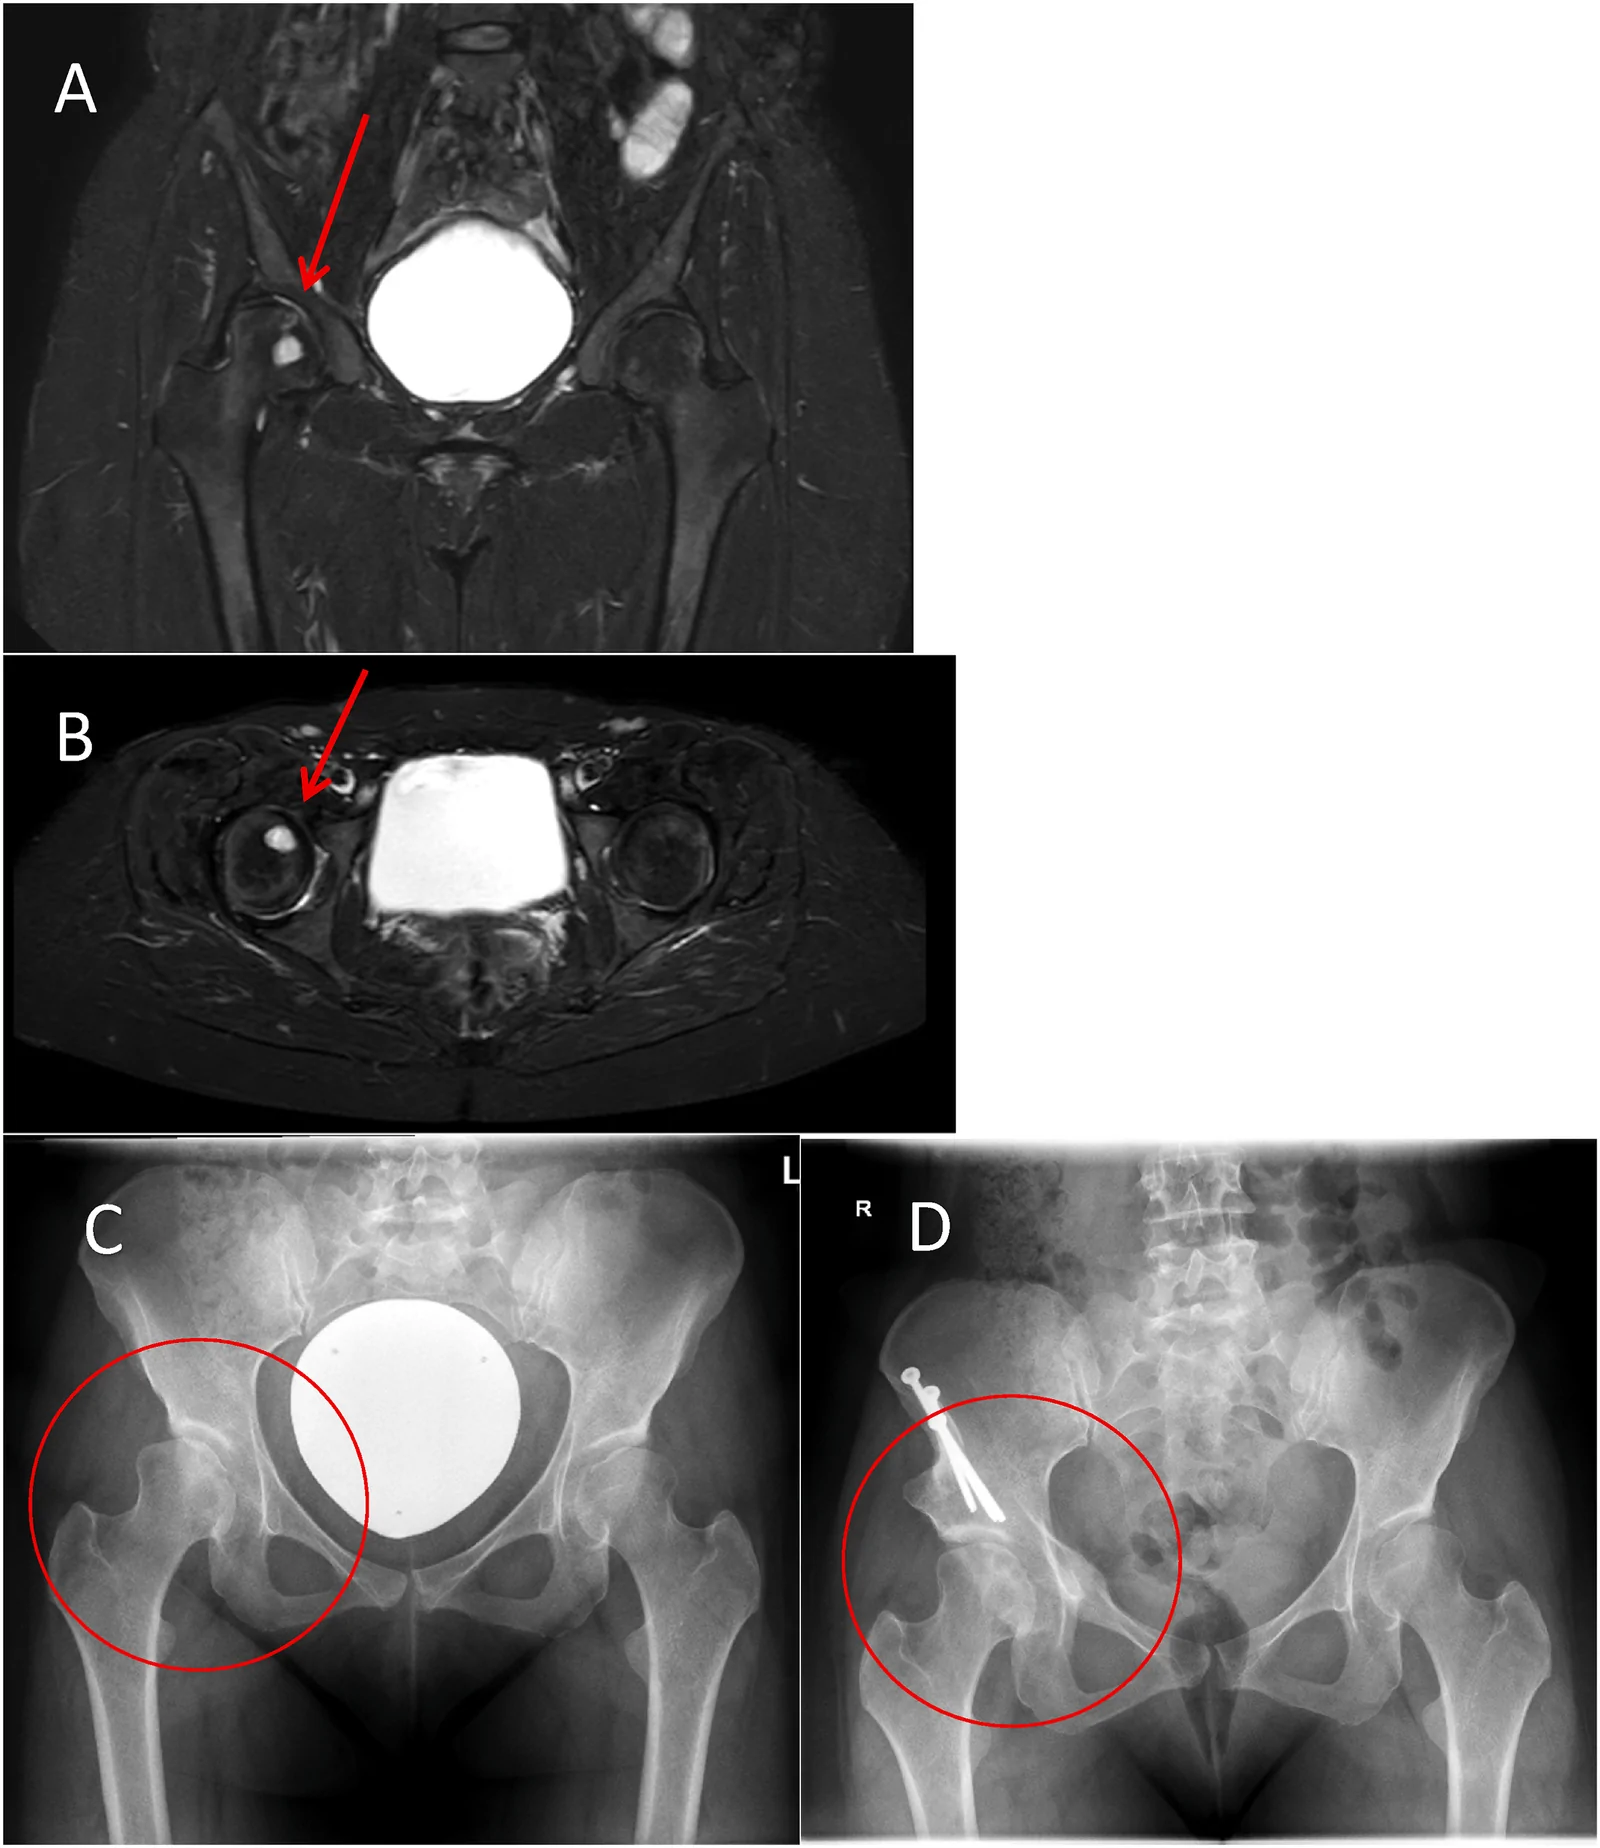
Example of subchondral cyst in the preoperative MRI A) preoperative MRI, coronar plane B) preoperative MRI, transversal plane C) preoperative x-ray, a.p. pelvis D) 1-year postoperative x-ray, a.p. pelvis.
